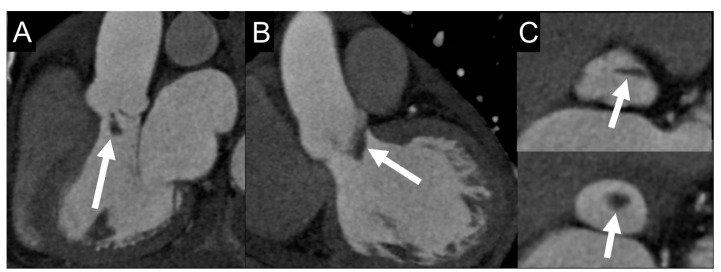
Vegetation on cardiac computed tomography angiography (CCTA) images. A 69-year-old man with fever and IE. Multiplanar CCTA reconstructions on three-chamber (A) and coronal (B) views show a 2-cm, hypodense, club-shaped, soft-tissue oscillating mass (arrows) attached to the ventricular side of aortic valve leaflets, which appear floating in the lumen of the left ventricular outflow tract in reconstructed axial valve planes (C). Sensitivity of CCTA in detecting vegetation ranges from 52.8% for small lesion to 94.4% for larger ones (>10 mm) [24].
